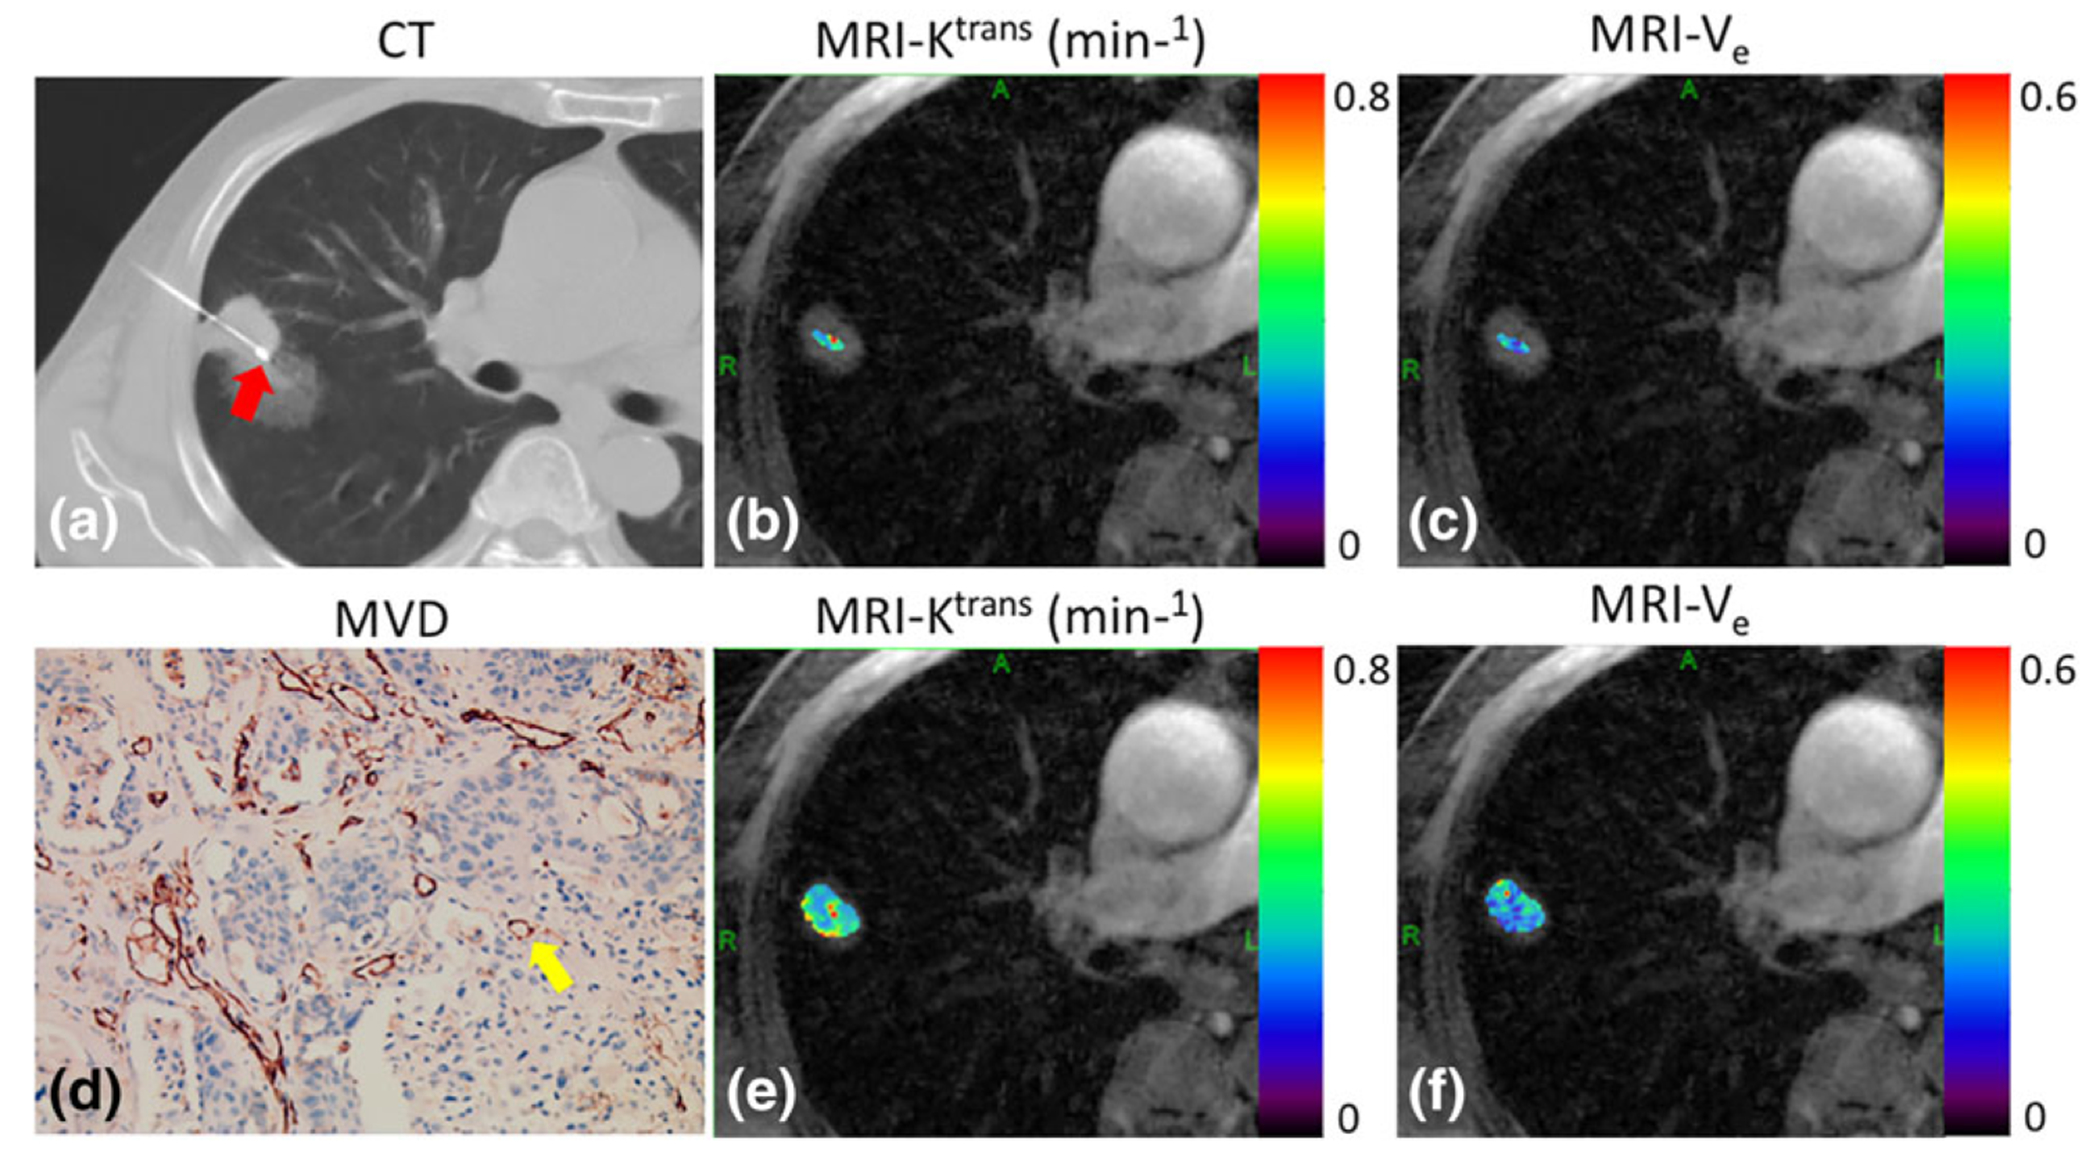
CT-biopsy image (a), MVD image (d) and both regional (b,c), and whole-tumor (e,f ) K^trans^ and V_e_ maps superimposed on a corresponding GRASP image from a patient with squamous cell carcinoma. The yellow arrow indicates a microvessel and the red arrow indicates the needle in CT biopsy.
